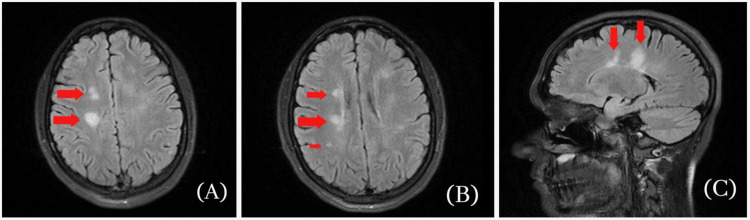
T2-weighted MRI images of the brain. A & B: Axial images of brain MRI demonstrating several foci of FLAIR hyperintensities (red arrows); C: Sagittal image of brain MRI showing large FLAIR in right posterior frontal lobe measuring 1.4 cm (red arrow). FLAIR: fluid attenuated inversion recovery
